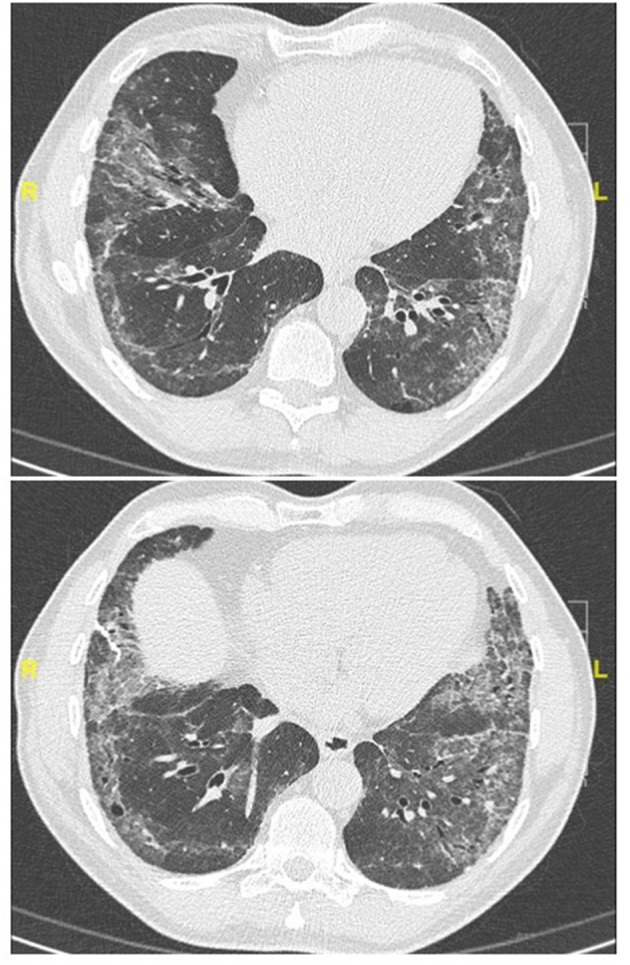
Chest CT (lung window) in a 62-year old, non-smoker male patient fulfilling IPAF criteria, with puffy fingers, mechanics' hands, gastro-esophageal reflux, high titer (1:1280) antinuclear antibodies. Lung biopsy demonstrated a pattern of probable usual interstitial pneumonia without fibroblastic foci and a paucity of lymphocytic inflammation (biopsy courtesy of Prof F. Thivolet-Béjui, Lyon).
